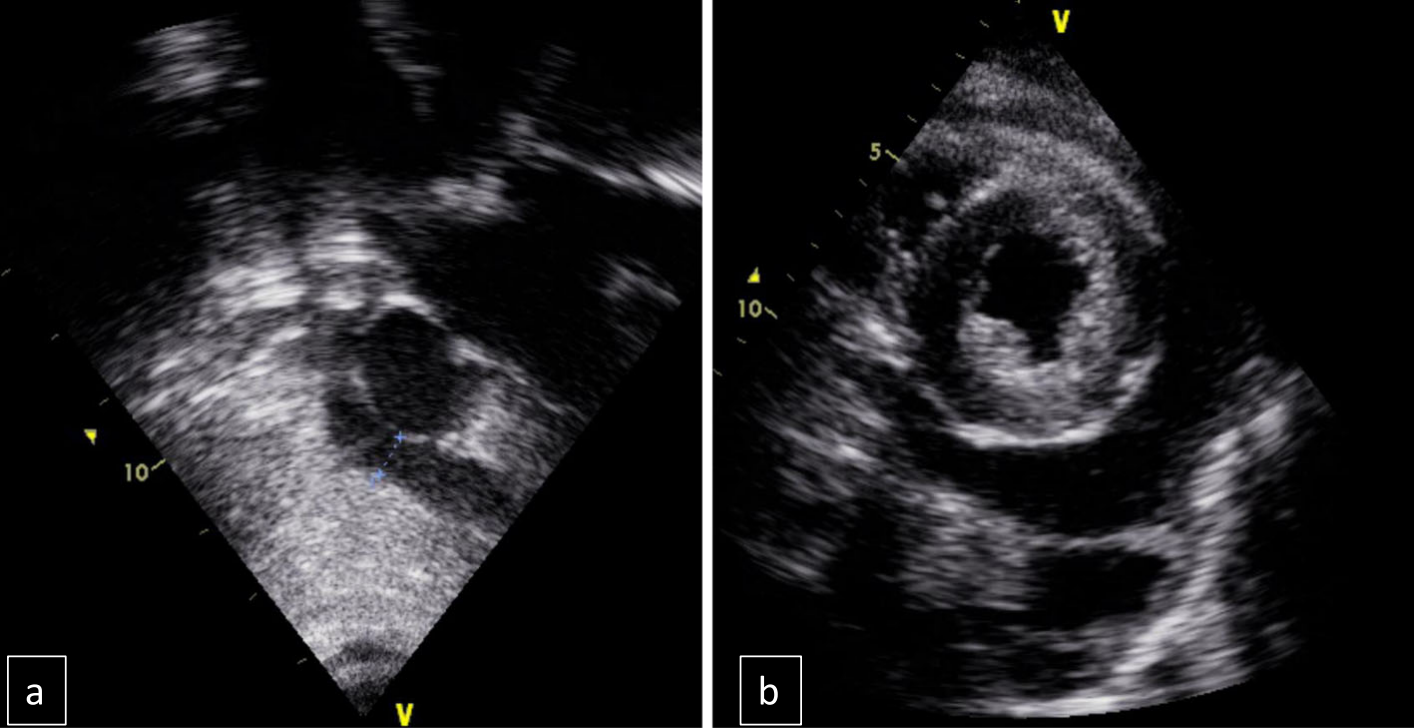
Echocardiography on admission to intensive care. a: pericardial effusion behind the right atrium. b: parasternal short axis view with a pericardial effusion
